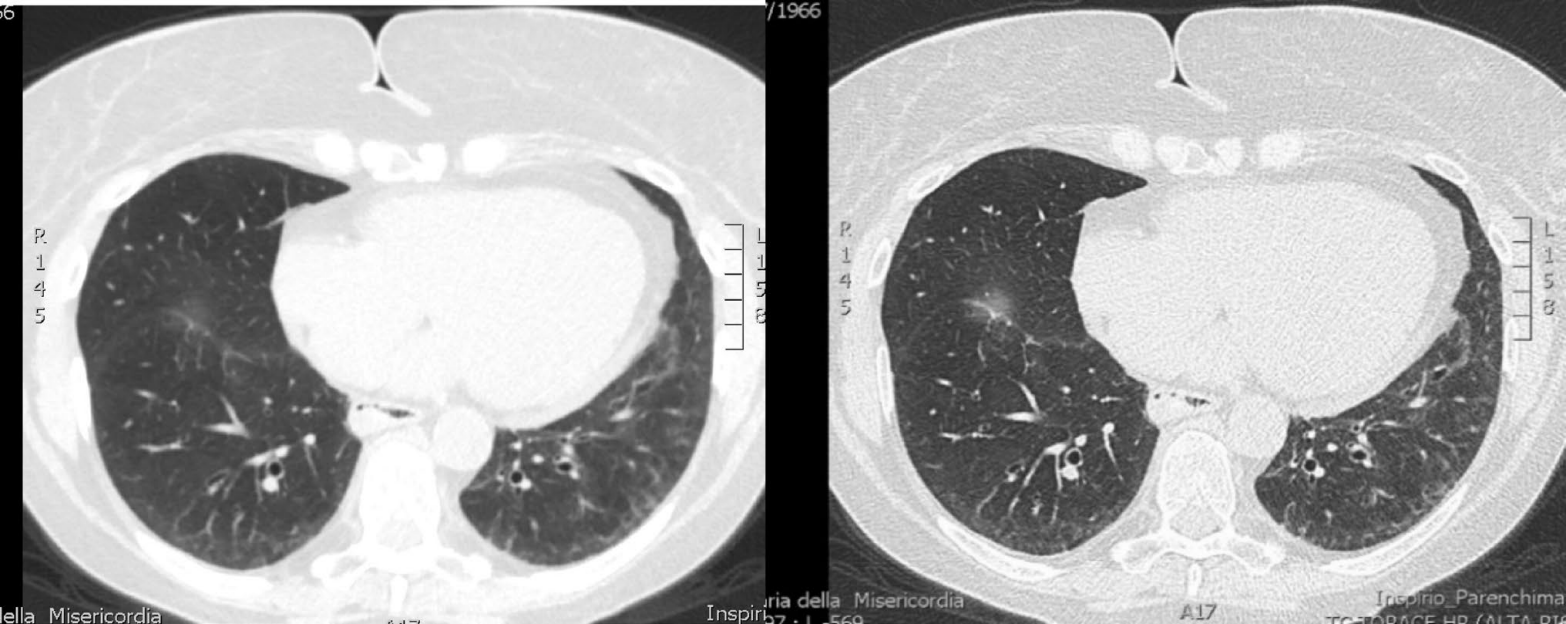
Follow-up HRCT showing a reduction in ground-glass opacities but persistent bilateral basal reticular pattern
